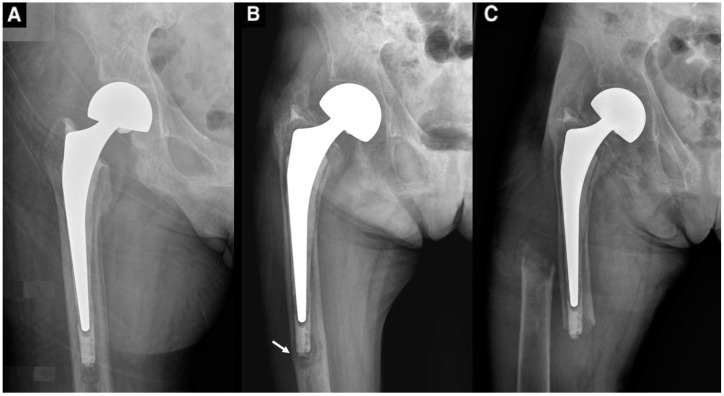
Serial X-rays showing the progression of femoral stem loosening. (A) An X-ray immediately following arthroplasty shows inadequate bone-to-cement fixation. (B) The progressive loosening of the implant 9 months following the index surgery, with impending fracture (narrow arrow), and (C) the resultant periprosthetic fracture at the tip of the cement 12 months following arthroplasty. Notice the thinning of the cortex over time.
